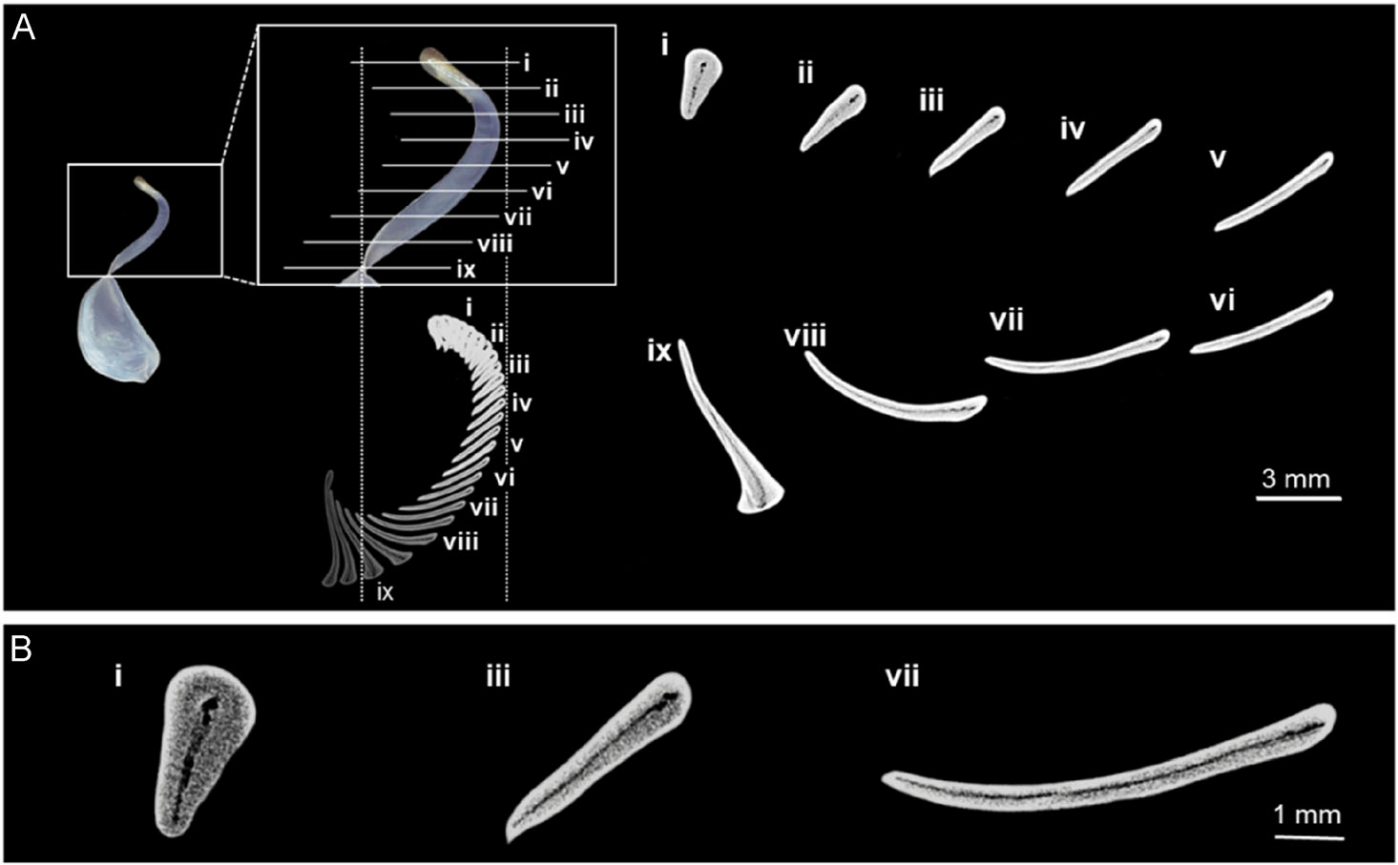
Photo and X‐ray CT images of a clausilium stalk. A) transverse section images at multiple locations i–ix) in a stalk. i) Closest to the junction where it joins the main shell structure. B) Magnified views of i), iii), and vii).
